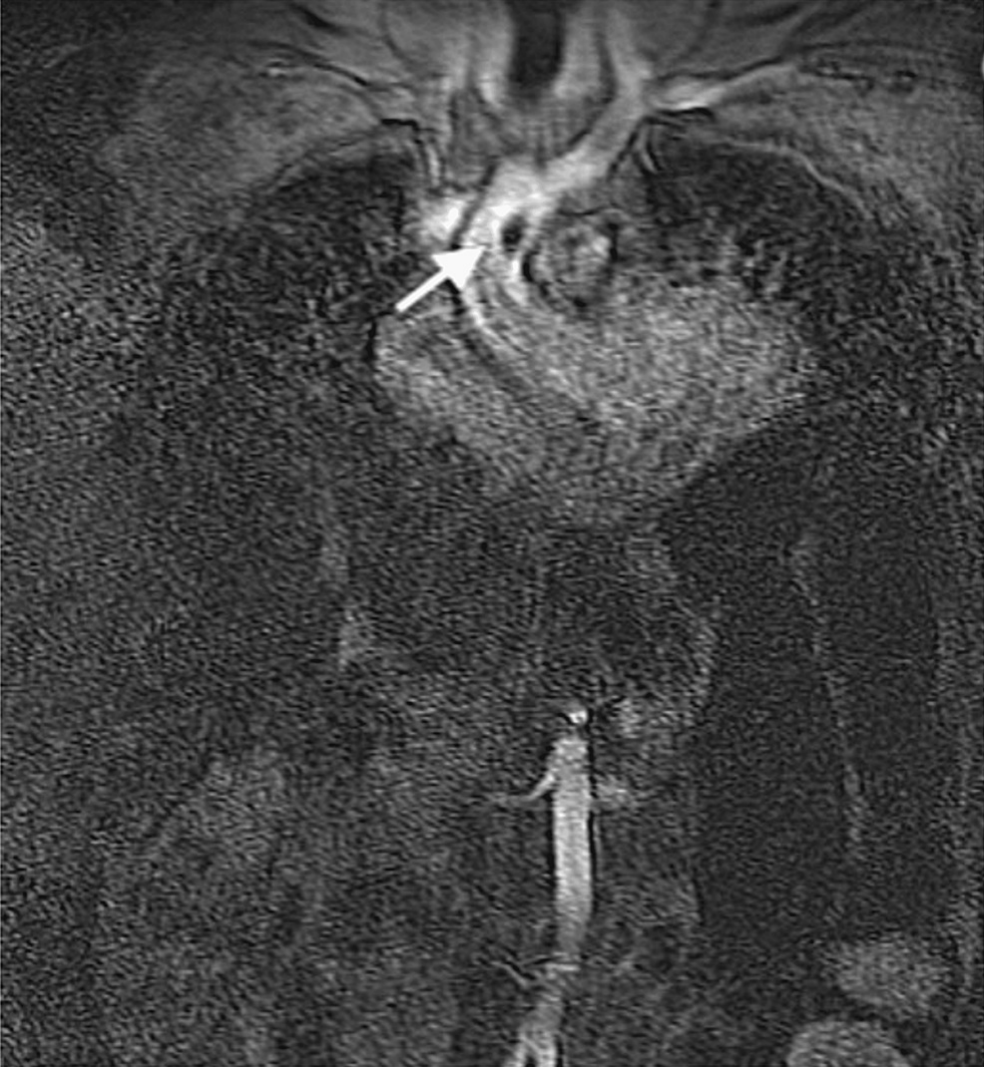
Contrast-enhanced magnetic resonance imaging showing an intraluminal filling defect (white arrow) in the mid ascending aorta with normal aortic size and wall thickness.
